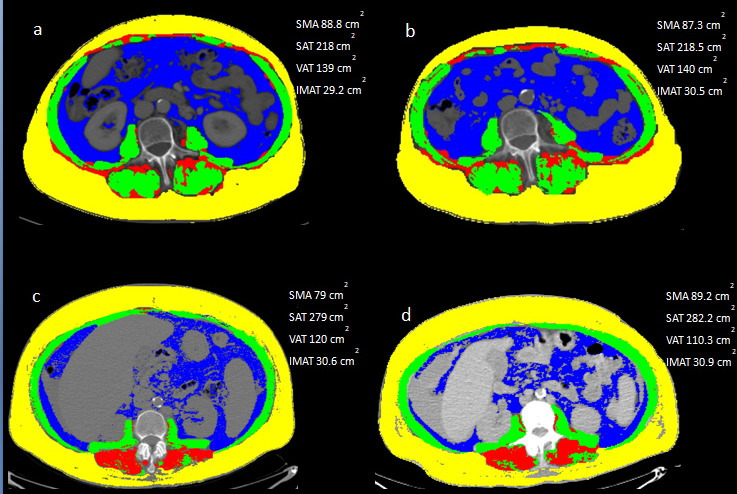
A representative case of measurements of SAT (yellow), VAT (blue), IMAT (red) and SMA (green) using HDCT (a) and LDCT (b) images. Another example with the comparison between HDCT (c) and LCT (d). HDCT, high-dose computedtomography; IMAT, intramuscular adiposetissue; LDCT, low-dose CT; SAT, subcutaneous adiposetissue; SMA, skeletal muscle area; VAT, visceral adipose tissue.
